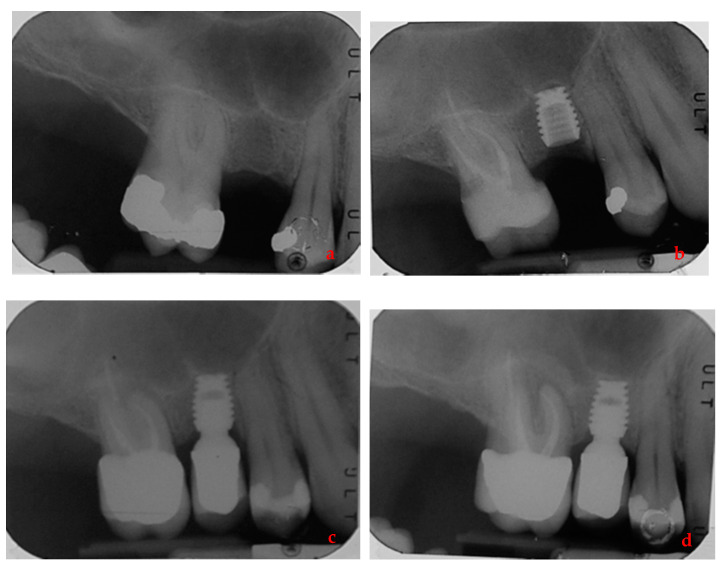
Single implant placed in 1.5 site (4.5 × 6 mm) of a male patient with history of periodontal disease: (a) pre-operative radiograph before implant placement; (b) radiograph obtained at implant placement; (c) radiograph obtained at time of loading; (d) radiograph obtained at 3-year follow-up.
